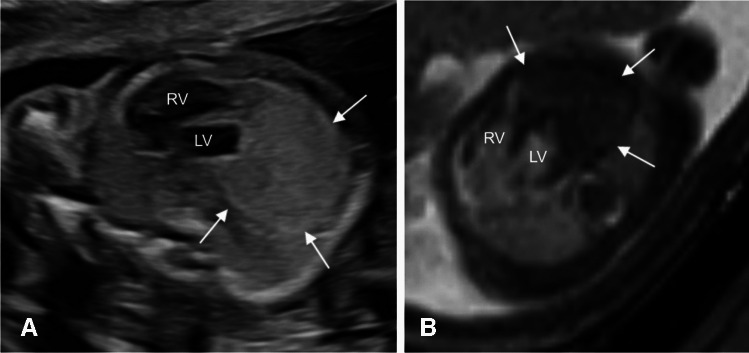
A fetal cardiac mass detected in a 20-week gestational age pregnancy. A Horizontal view echocardiogram demonstrating a large, well-defined hyperechoic mass at the cardiac apex (*arrows*). B Axial T2-weighted image shows a large solitary T2 hypointense mass (*arrows*). No additional lesions were seen. Imaging of fetal brain and kidneys was unremarkable. The differential considerations were fibroma or rhabdomyoma. Unfortunately, the pregnancy resulted in fetal demise, with genetic testing positive for tuberous sclerosis and an autopsy confirming a rhabdomyoma. *LV*, left ventricle; *R* right ventricle
